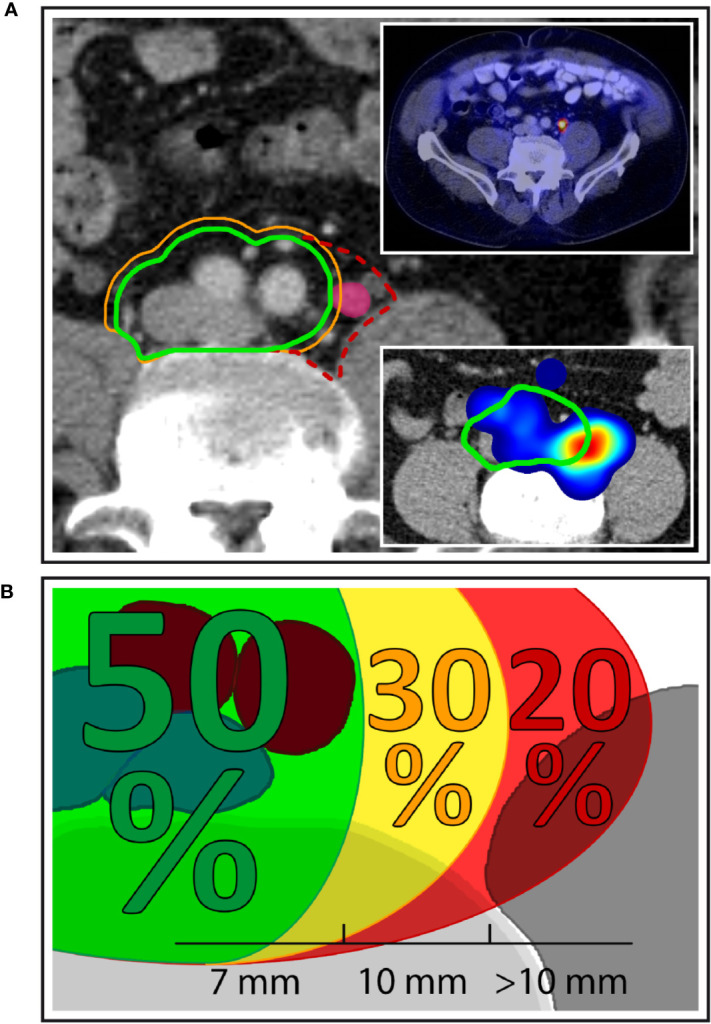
Left common iliac nodal metastases outside NRG consensus CTV. (A) Exemplary patient case with a left common iliac nodal metastasis outside the NRG CTV recommendation using margin options of 7 mm (green contour) and 10 mm (orange contour), respectively. Dashed red contour: proposed extension of the CTV to the medial surface of the left major psoas muscle. Top right inset: PSMA-PET/CT of the same patient demonstrating PSMA uptake. Bottom right inset: Corresponding anatomic location in the independent mapping analysis showing a high-probability hotspot that extends beyond the left boundary of the NRG CTV recommendation. (B) Overall, 50.0% of patients with left common iliac nodal involvement had all common iliac metastases covered using a margin of 7 mm around the vessels. A 10 mm margin encompassed all nodal metastases in an additional 30.0% of patients, while 20.0% of patients with left common iliac metastases had lymph node metastases that extended even beyond the 10-mm margin.
